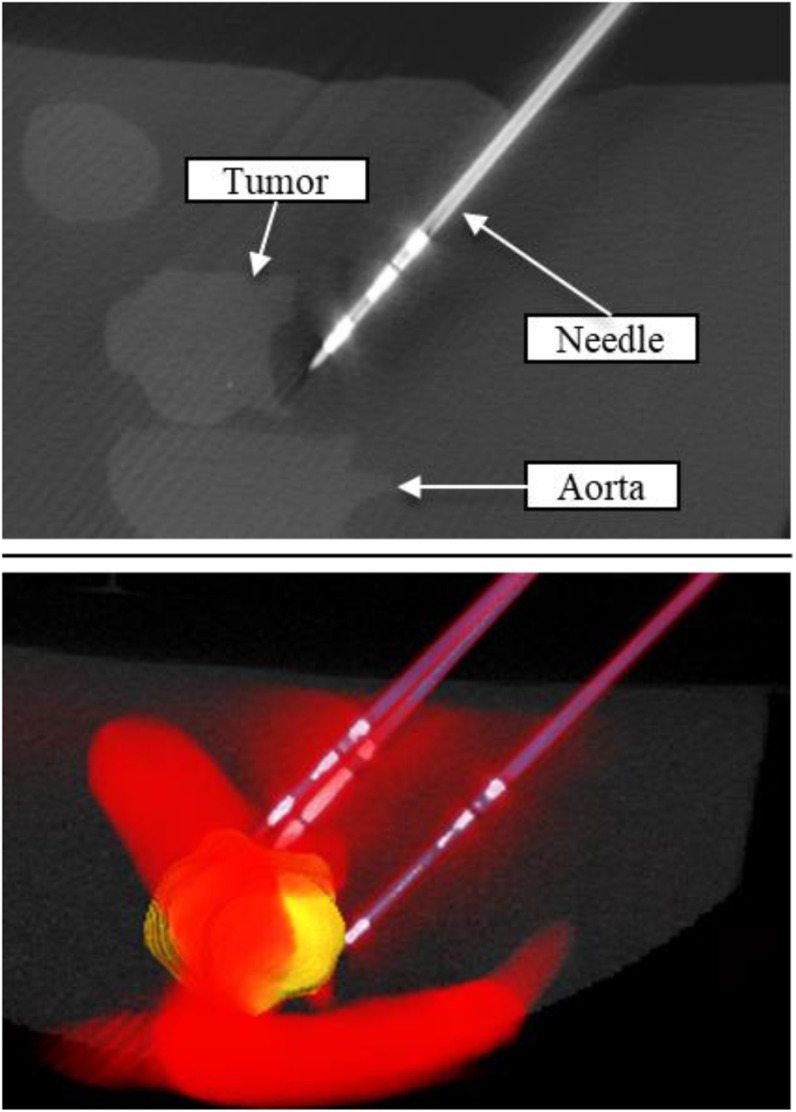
Placed ablation needles around tumour. CT slice (top), volume rendering (bottom).
